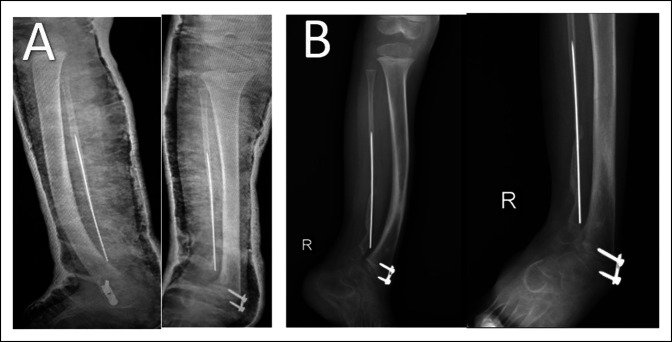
After 4 weeks, we saw callus formation in the radiograph (A); in the 3-month postoperative radiograph, we saw full union in the congenital pseudarthrosis of the fibula site (B).
